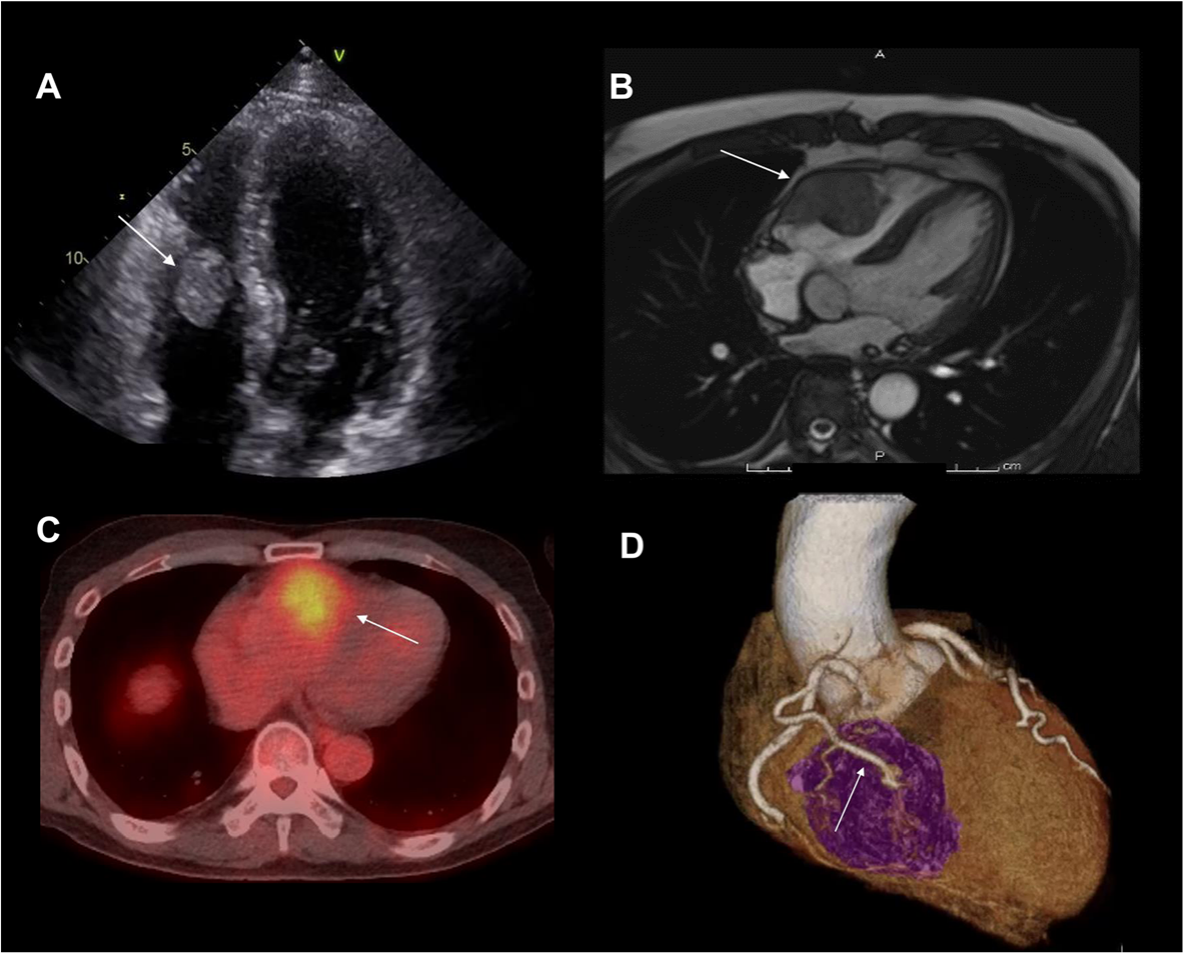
a Transthoracic echocardiography shows a 52 × 31 mm right ventricular mass (arrow) moving without extension into the outflow tract. b Cardiac MRI showed an anterior right ventricular free wall mass of the RV myocardium. c Axial fused FDG-PET/CT image demonstrates mildly FDG-avid mass within right ventricular wall (arrow). d A cardiac CT angiogram (segmented three-dimensional volume rendered image) shows the RV branches entering (arrow) the mass
